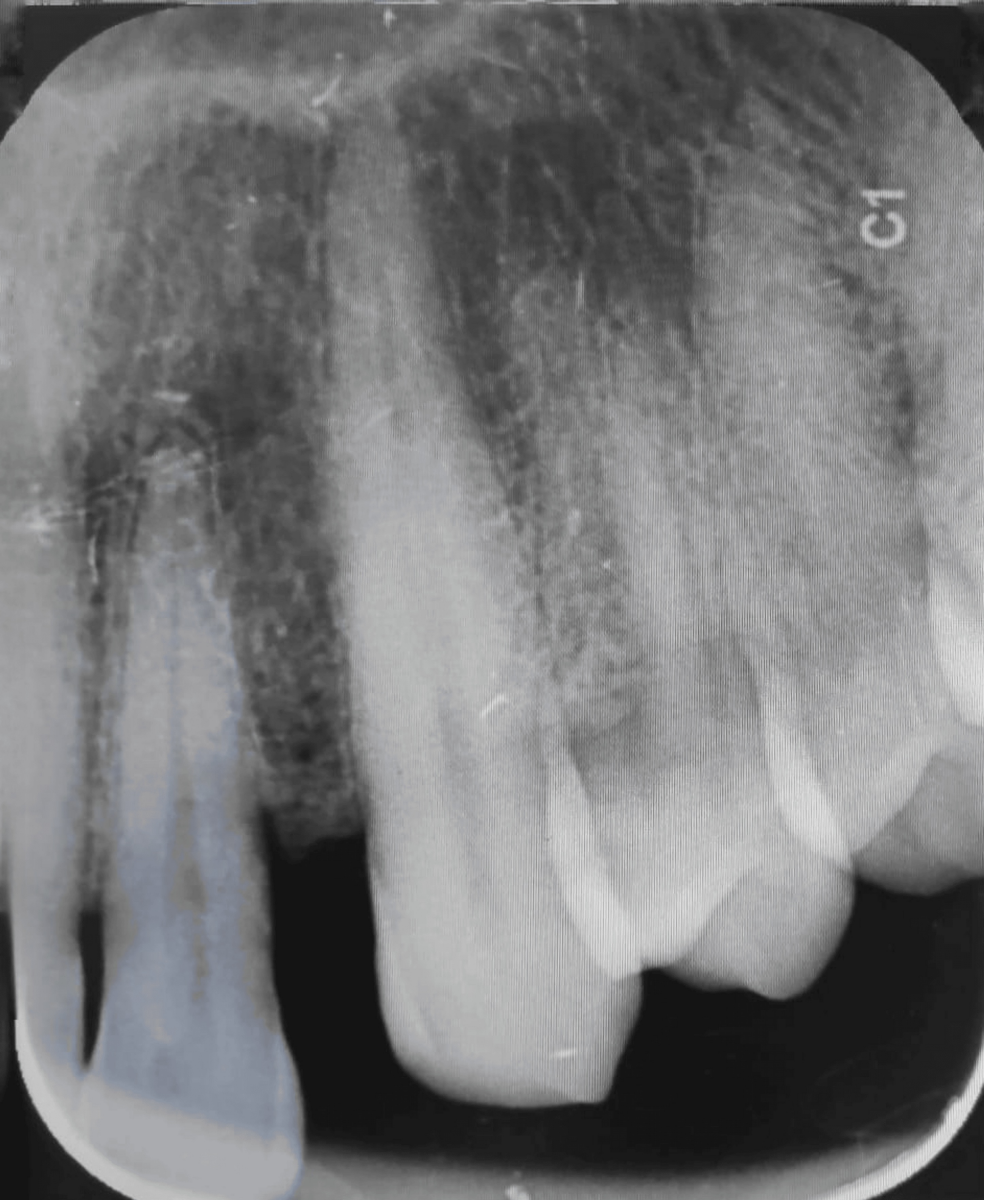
An intraoral periapical radiograph of the area affected by the lesion
